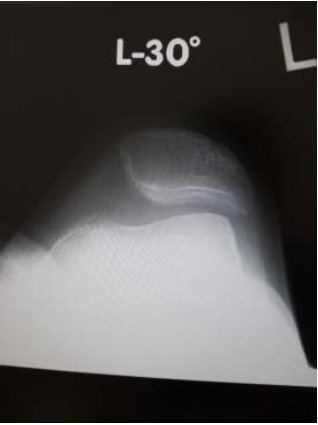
Roentgenogram showing lateral patellar subluxation.
